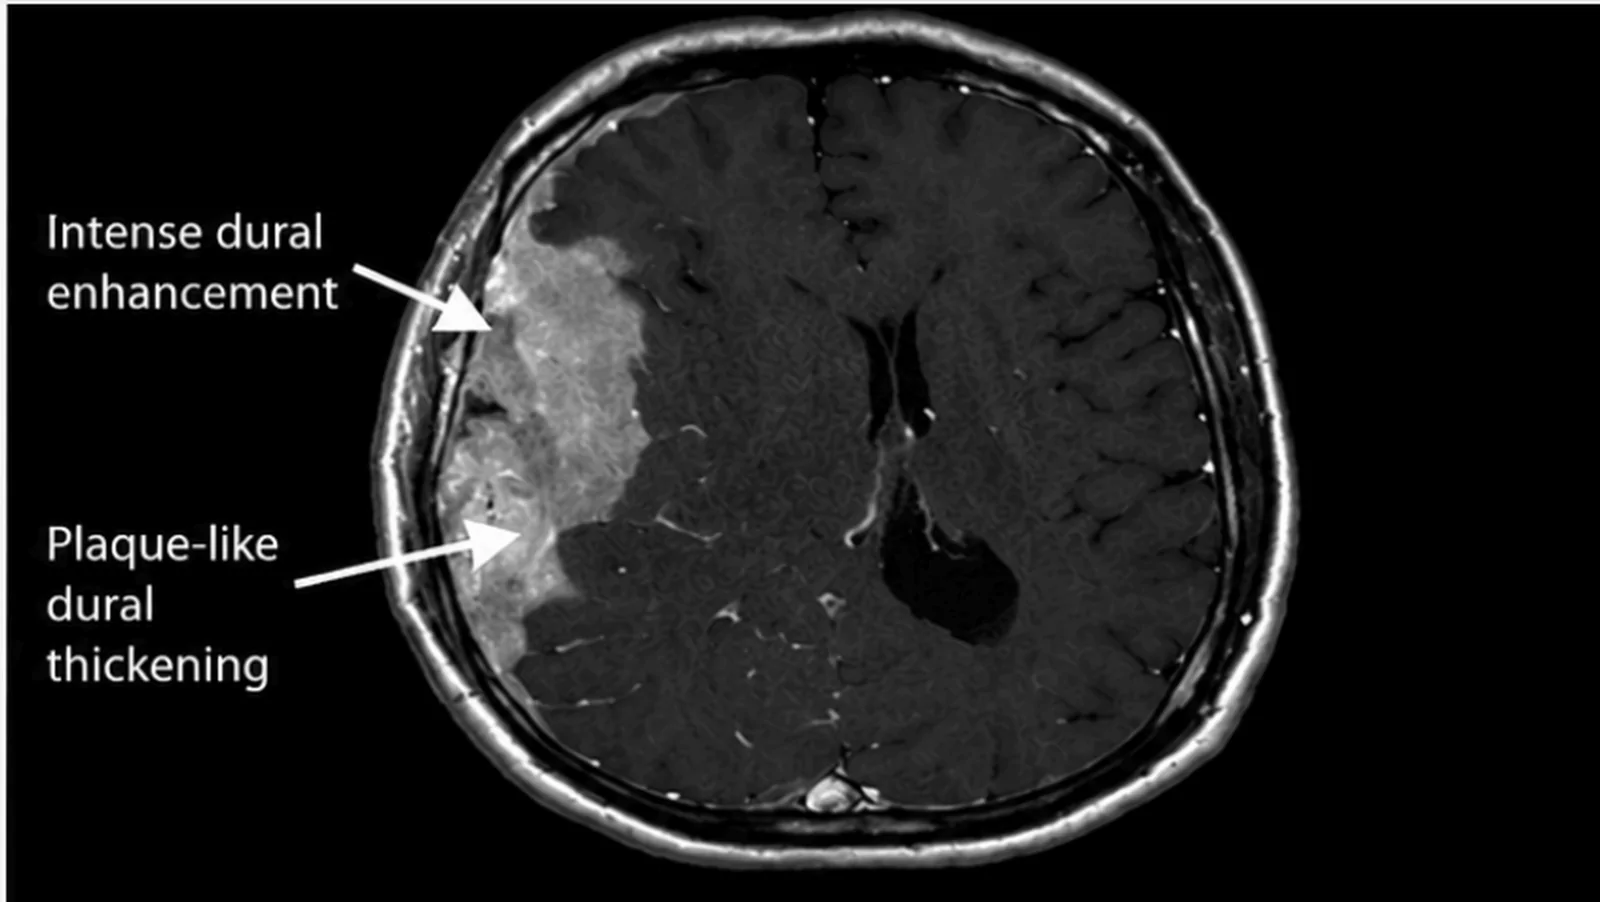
Preoperative contrast-enhanced T1-weighted MRI. Axial contrast-enhanced T1-weighted magnetic resonance imaging (MRI) demonstrating intense dural enhancement (white arrow) and plaque-like dural thickening (arrowhead), radiologically mimicking a meningioma en plaque.
